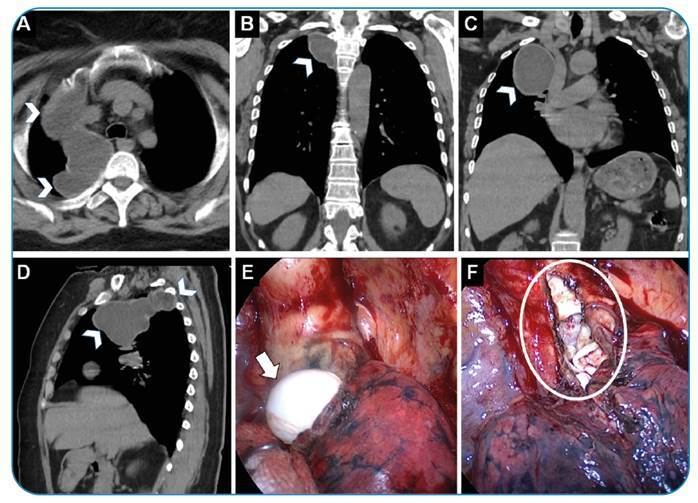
(A) Axial, (B, C) coronal, and (D) sagittal thoracic computed tomography sections show a mediastinal cystic lesion of approximately 10 x 4 cm (arrowheads) extending from the apex to the perihilar area in the right hemithorax. (E, F) The lesion was diagnosed intraoperatively as a hydatid cyst (arrow, laminated membrane; circle, cyst cavity).
